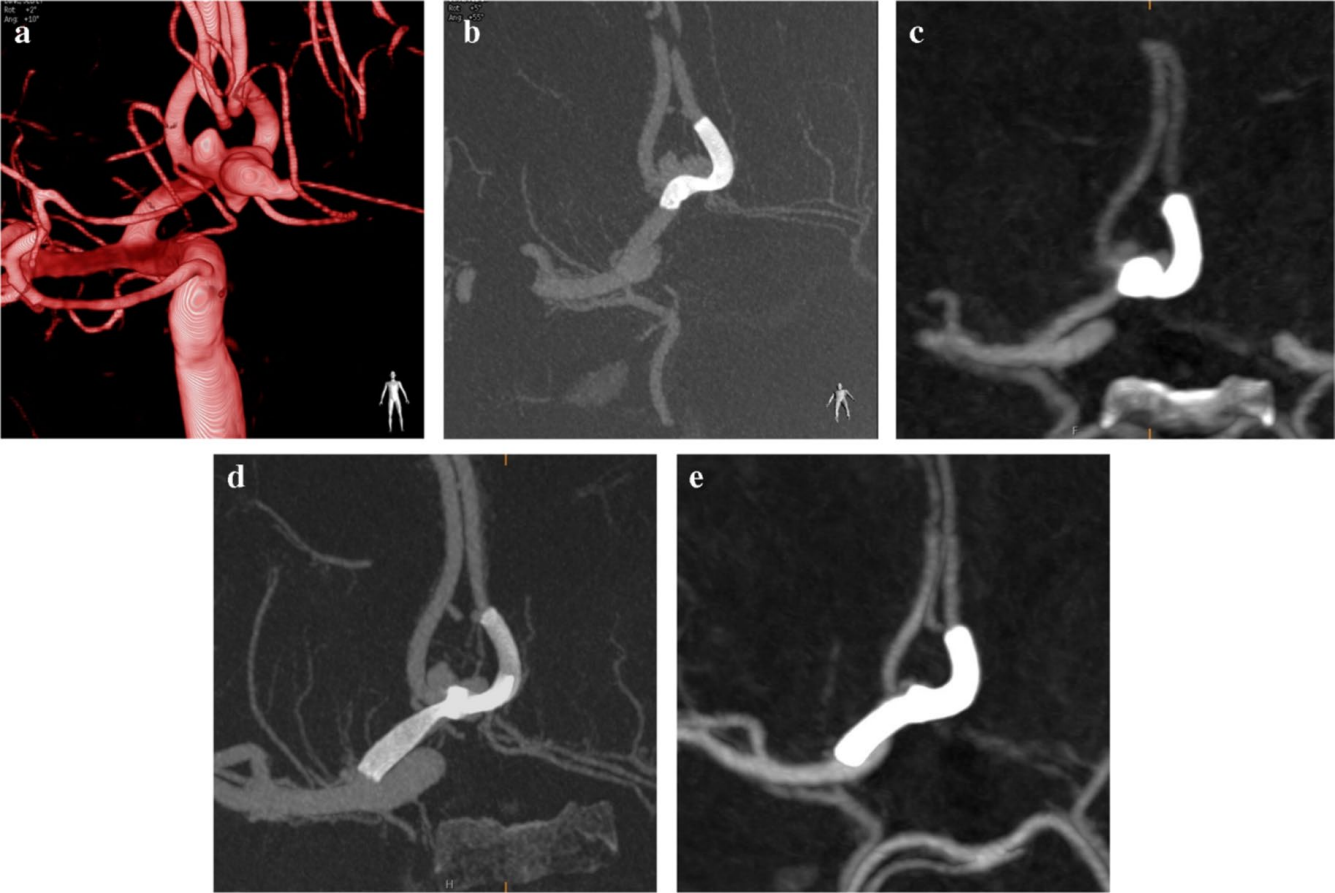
A 49-year-old male patient presented with an incidental saccular aneurysm. (a). A frontal 3D angiogram demonstrated a multilobulated saccular aneurysm arising from the ACom artery. (b) A cone-beam CT scan obtained after SVB stent implantation. (c) A six-month follow-up CTA revealed proximal shortening and deformation of the stent, along with contrast filling of the aneurysm. (d) A second SVB stent was subsequently implanted, and a cone-beam CT scan obtained after the procedure clearly demonstrated proximal deformation of the first stent and suboptimal expansion of the second stent. Fifteen months after the second treatment, follow-up CTA revealed some residual filling of the aneurysm, although marked shrinkage was evident
